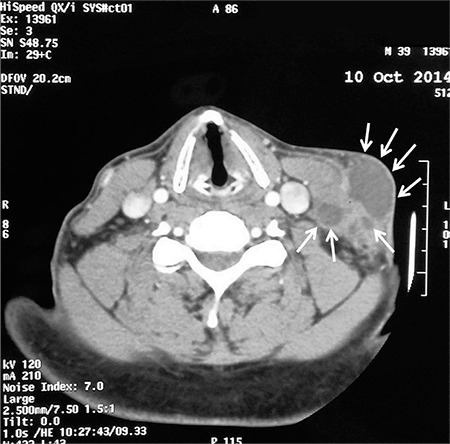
Axial CT scan of the neck shows multiple cystic lesions on the left neck compatible with an abscess.
